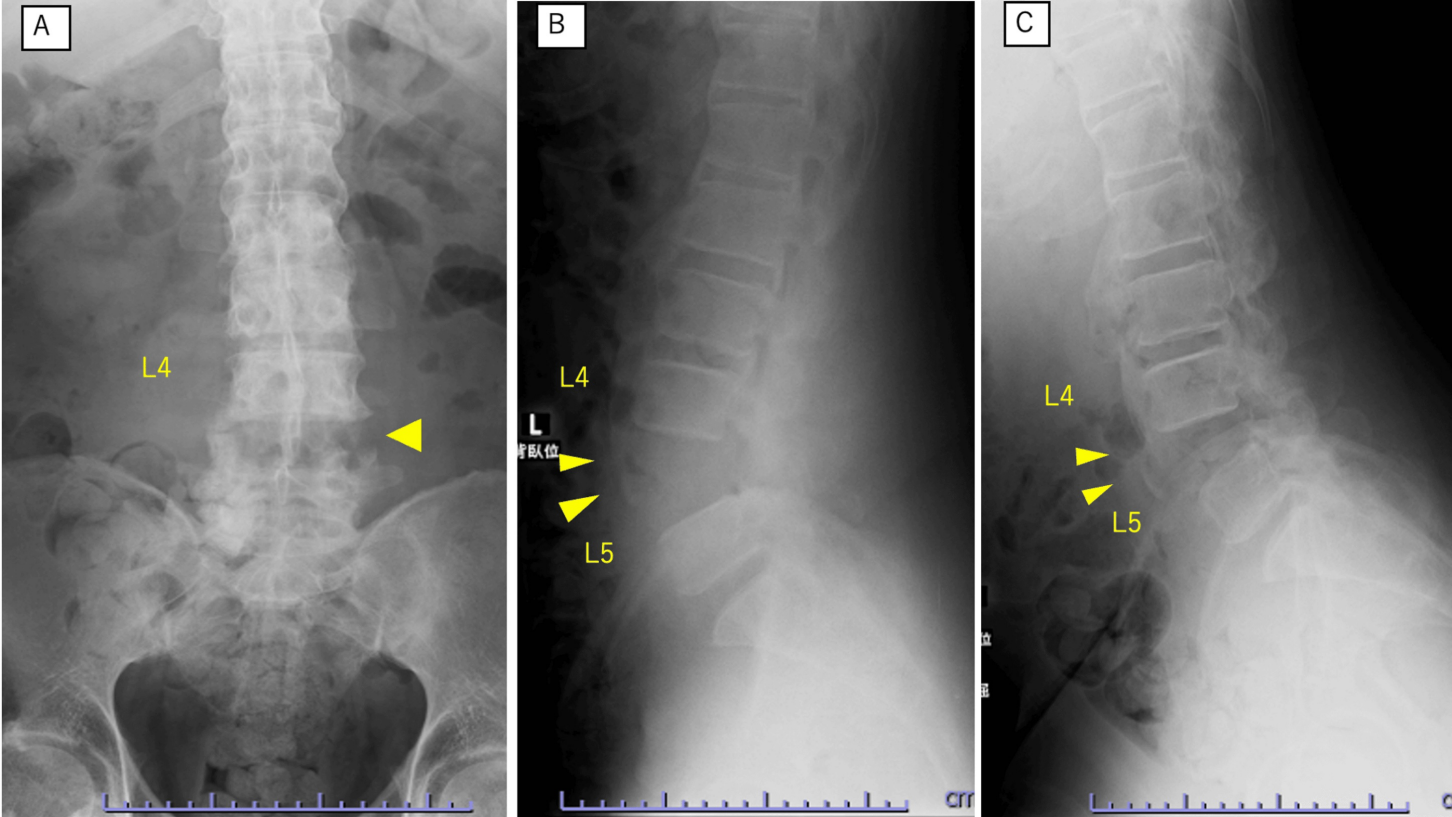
Preoperative dynamic radiographs demonstrating dislocation and instability at L4/L5. (A) Anteroposterior radiograph showing widening of the L4/L5 disc space (arrowheads). (B) Lateral radiograph obtained in the supine position showing widening of the L4/L5 disc space (arrowheads). (C) Lateral radiograph obtained in the lateral decubitus flexion position showing partial reduction of the disc space widening at L4/L5, indicating positional instability at the only remaining mobile segment (arrowheads).
